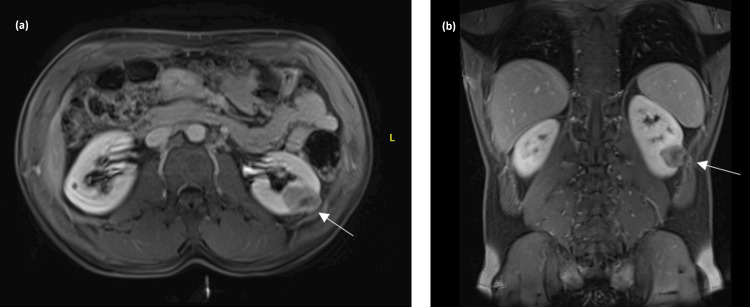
Abdominal MRI. (a) Axial T1 VIBE (volumetric interpolated breath-hold examination) and (b) coronal T1 VIBE showing a left renal exophytic lesion measuring 2.4 × 3.1 cm and demonstrating iso-/hyperintense T2 signal, isointense T1 signal, with diffusion restriction and faint enhancement, with no fat component or signal drop on in- and out-of-phase imaging.
